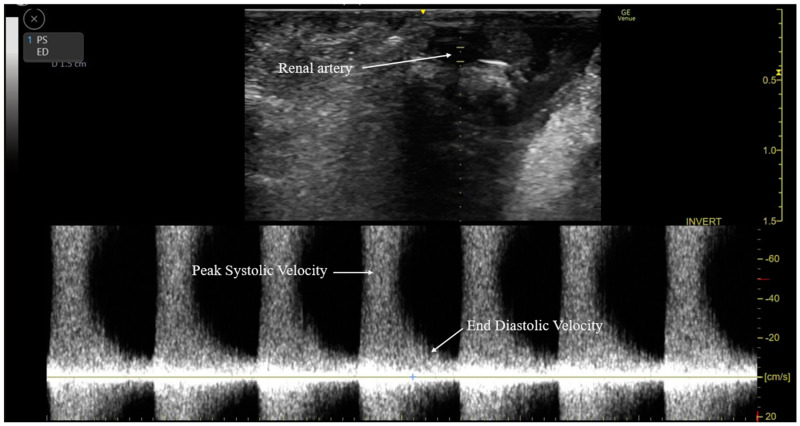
Measurement of renal arterial resistive index by using pulsed wave Doppler ultrasonography.
